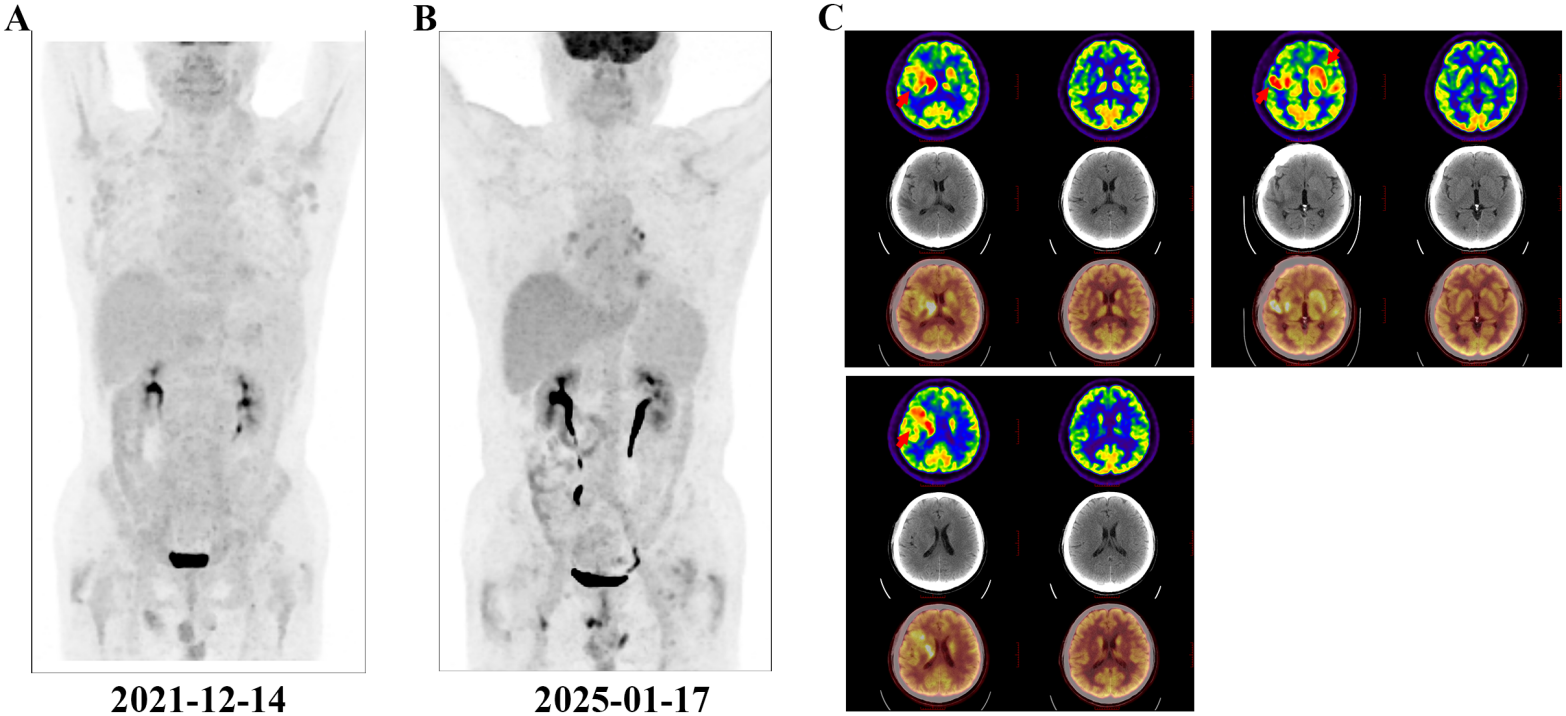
PET-CT examination. The red arrows indicate the intracranial involved lesion. (A) Multiple systemic foci when diagnosing CLL; (B) Disappearance of systemic foci when diagnosing DLBCL; (C) Lesions of isolated intracranial involvement when diagnosing DLBCL.
